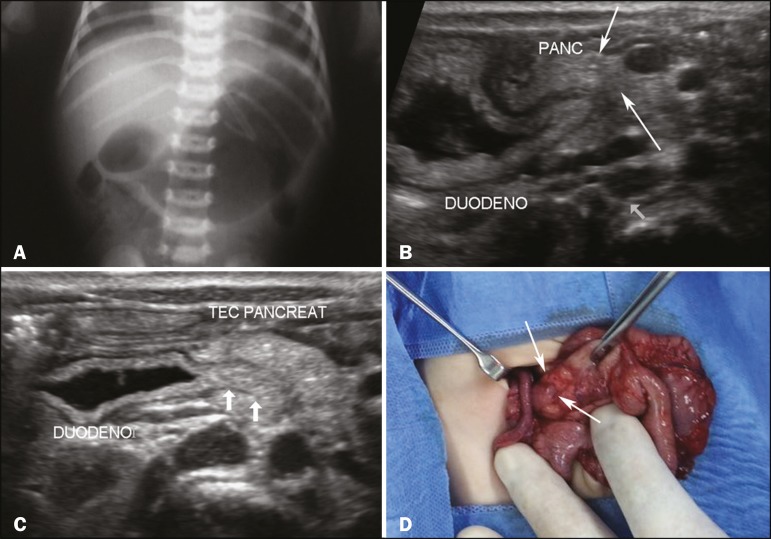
A: X-ray of the abdomen, showing gas distention of the stomach
